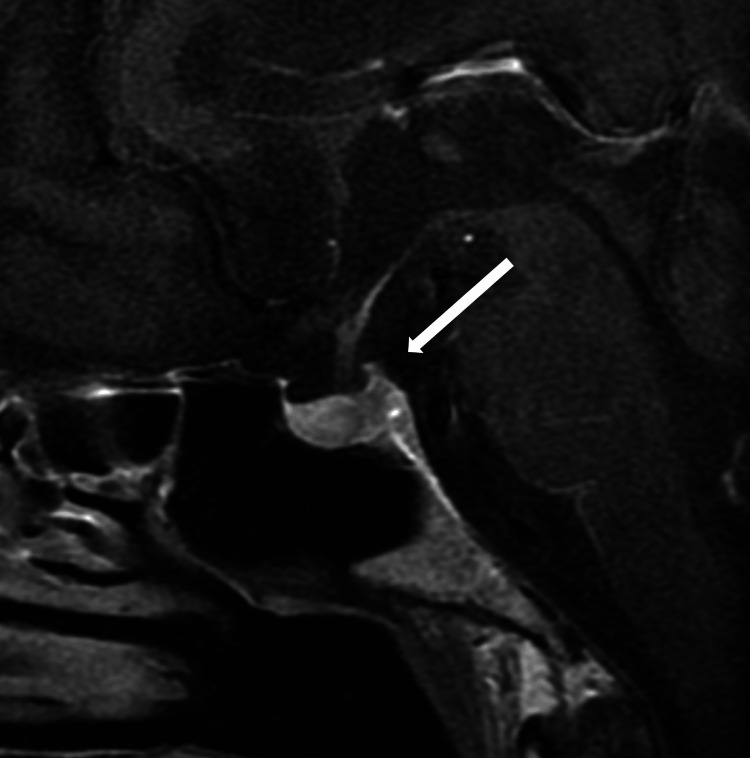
A T1-weighted sagittal image of the pituitary on MRI. Pituitary MRI showed no noticeable change.
